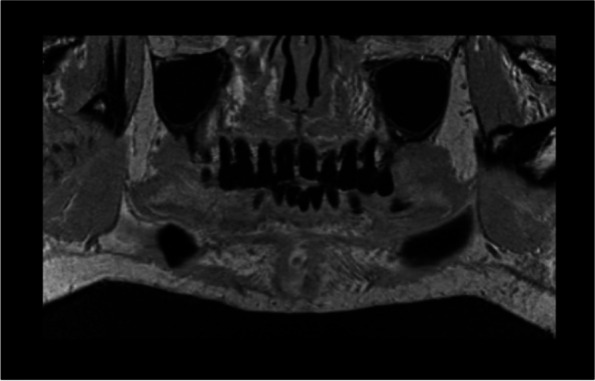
Preoperative MRI before vertical bone augmentation, coronal T1 weighted showing no pathological findings
